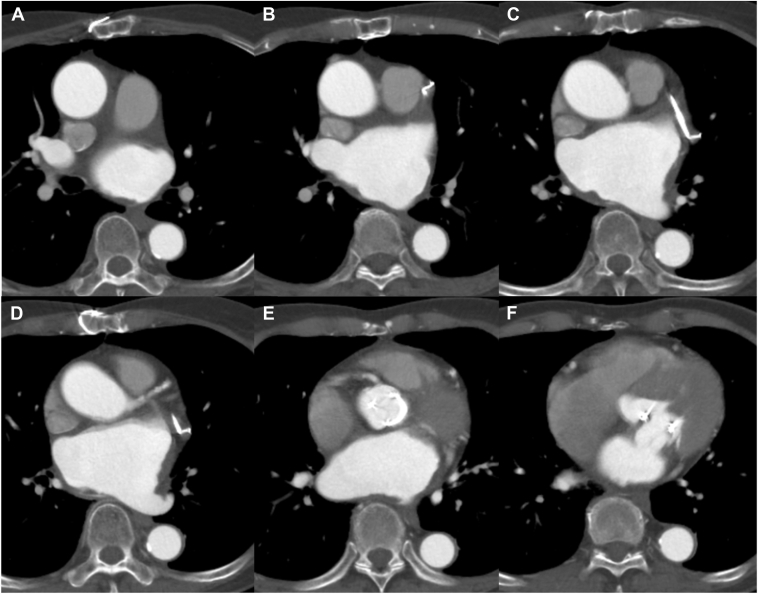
The computed tomography images obtained 3 months later revealed partial thrombus remnants on the lateral and posterior walls, but a reduction in thrombus size was observed.
